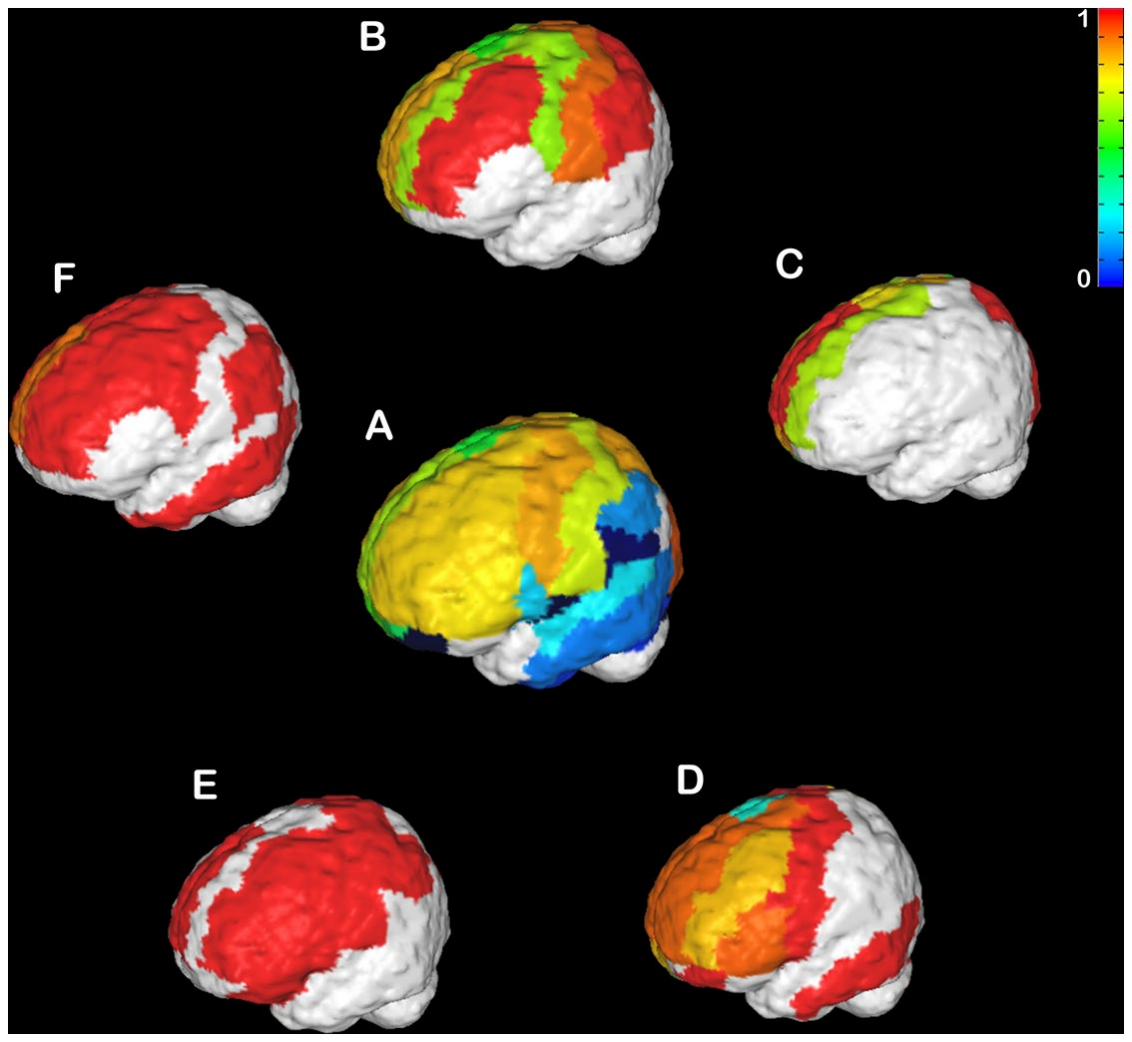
Individual Heterotopicity map of hypoplasic CCD subjects. DWI callosal tractography-based heterotopicity maps of the populational average typical subject (A) and each hypoplasic CCD subject (B–D). The color bar represents the scale of heterotopicity, with cool colors showing homotopic areas and hot colors showing heterotopic areas. The individual maps reveal a large individual variance of each subject relative to the average map of typical individuals.
